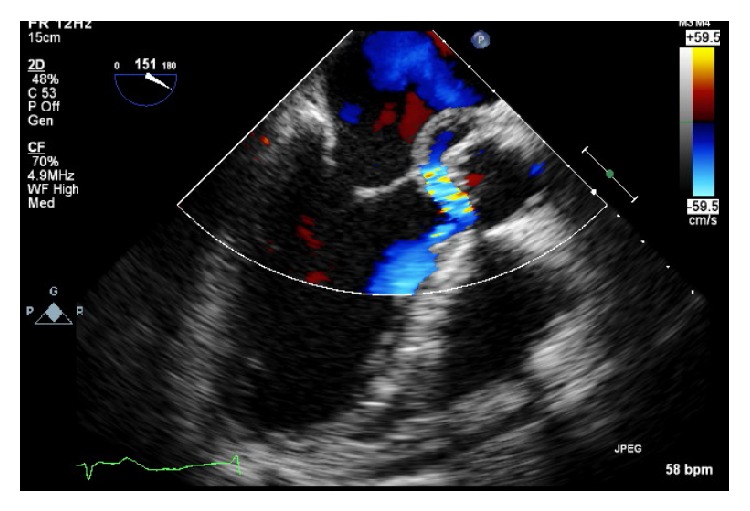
Transesophageal echocardiogram at midesophageal long-axis view with Doppler revealing regurgitation into abscess surrounding the aortic valve suggestive of aortic fistula.
